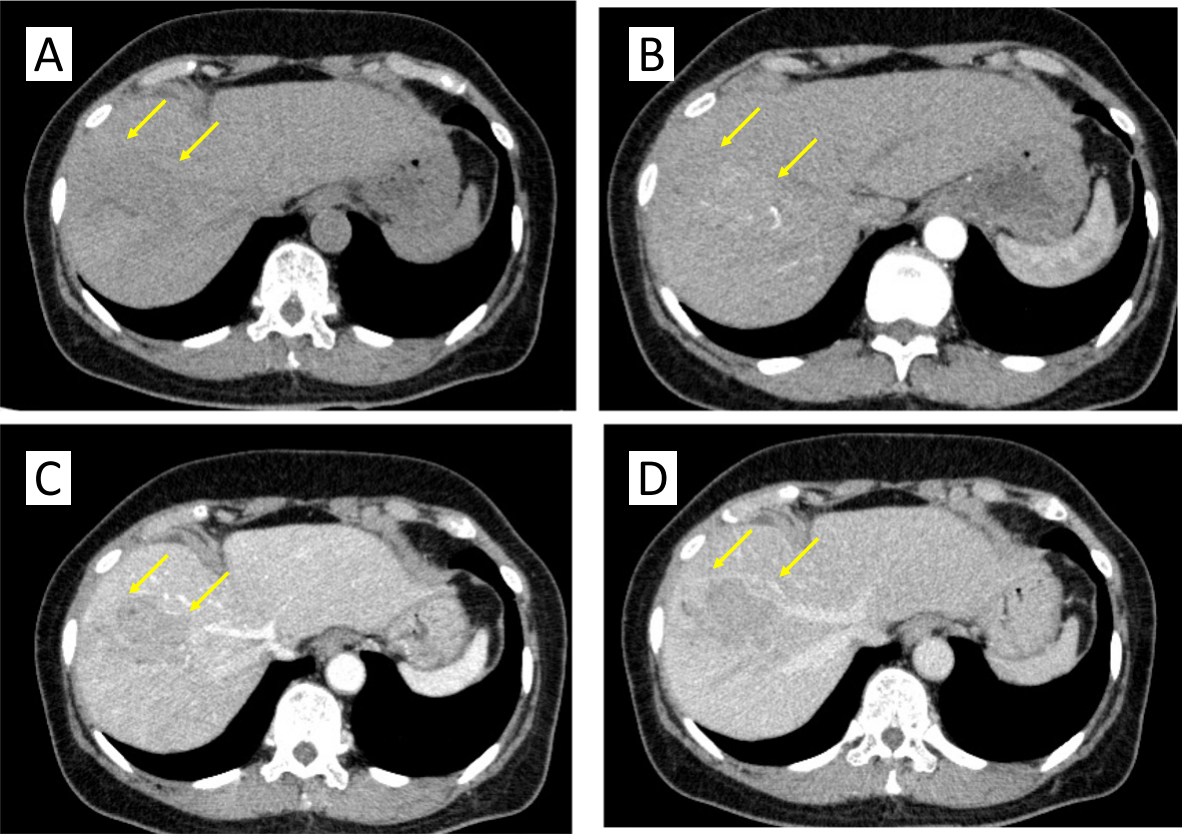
Preoperative dynamic enhanced computed tomography. A Plain image. B Arterial phase. C Portal phase. D Equivalent phase. A 6-cm early enhanced, unclear boundary tumor was seen in the anterior section of the liver, segment 8, which infiltrated the middle hepatic vein
